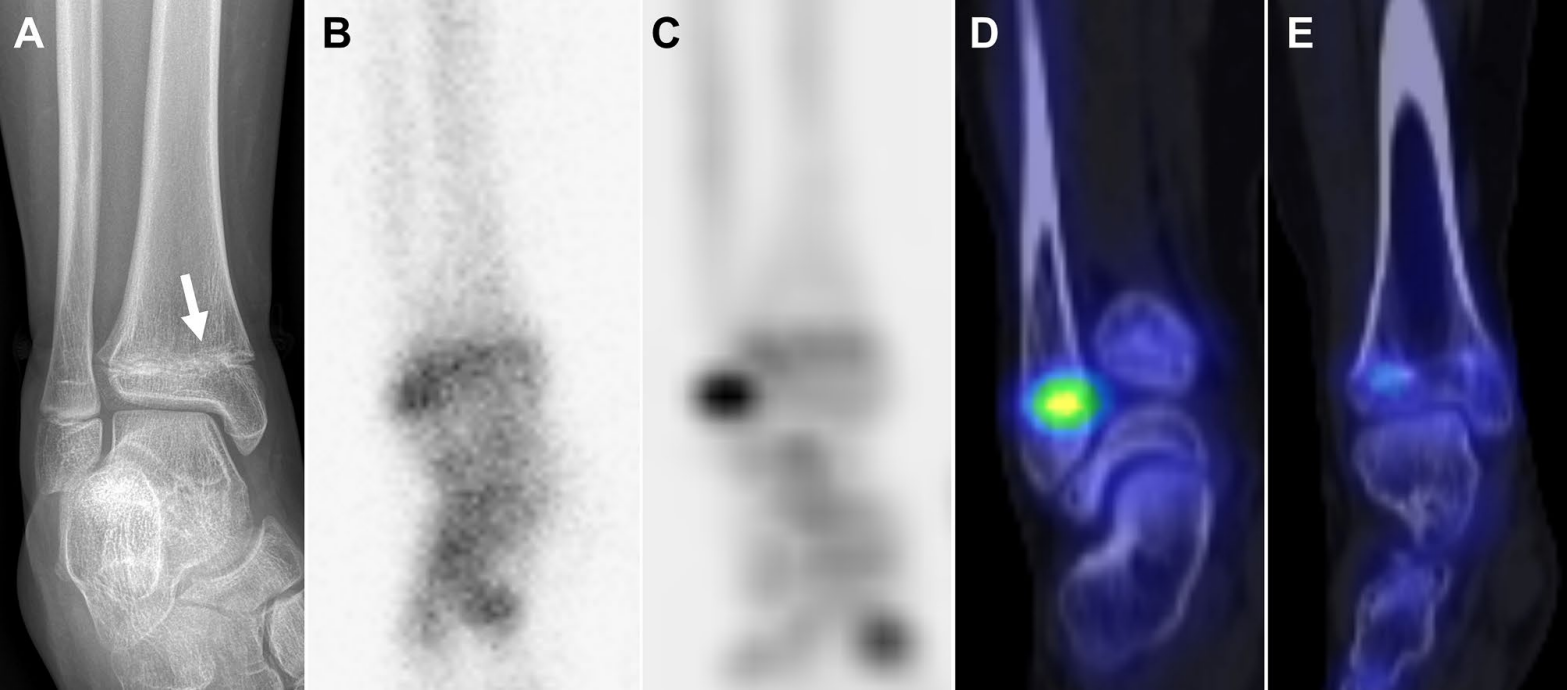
Advantage of bone SPECT/CT for assessing overlapping lateral tibial and fibular physes. A bone bridge (arrow) is suspected in the medial distal tibial physis on ankle mortise radiograph in an 11.4-year-old boy who sustained a physeal fracture (A). Bone SPECT/CT was performed to assess the growth potential in the remaining physis surrounding the bone bridge. On planar scintigraphy derived from SPECT/CT, the physeal activity of the distal tibia is unclear, due to the overlap of the distal fibular and lateral distal tibial physes (B). Differentiation of these two physes is possible on SPECT (C), although it is clearer on fused SPECT/CT (D and E). SPECT and SPECT/CT indicate physeal activity only in the distal fibular physis, whereas activity is absent in the near entire distal tibial physis. Based on these findings on SPECT/CT, epiphysiodesis was performed in the right distal fibula rather than bone bridge resection in the right distal tibia
